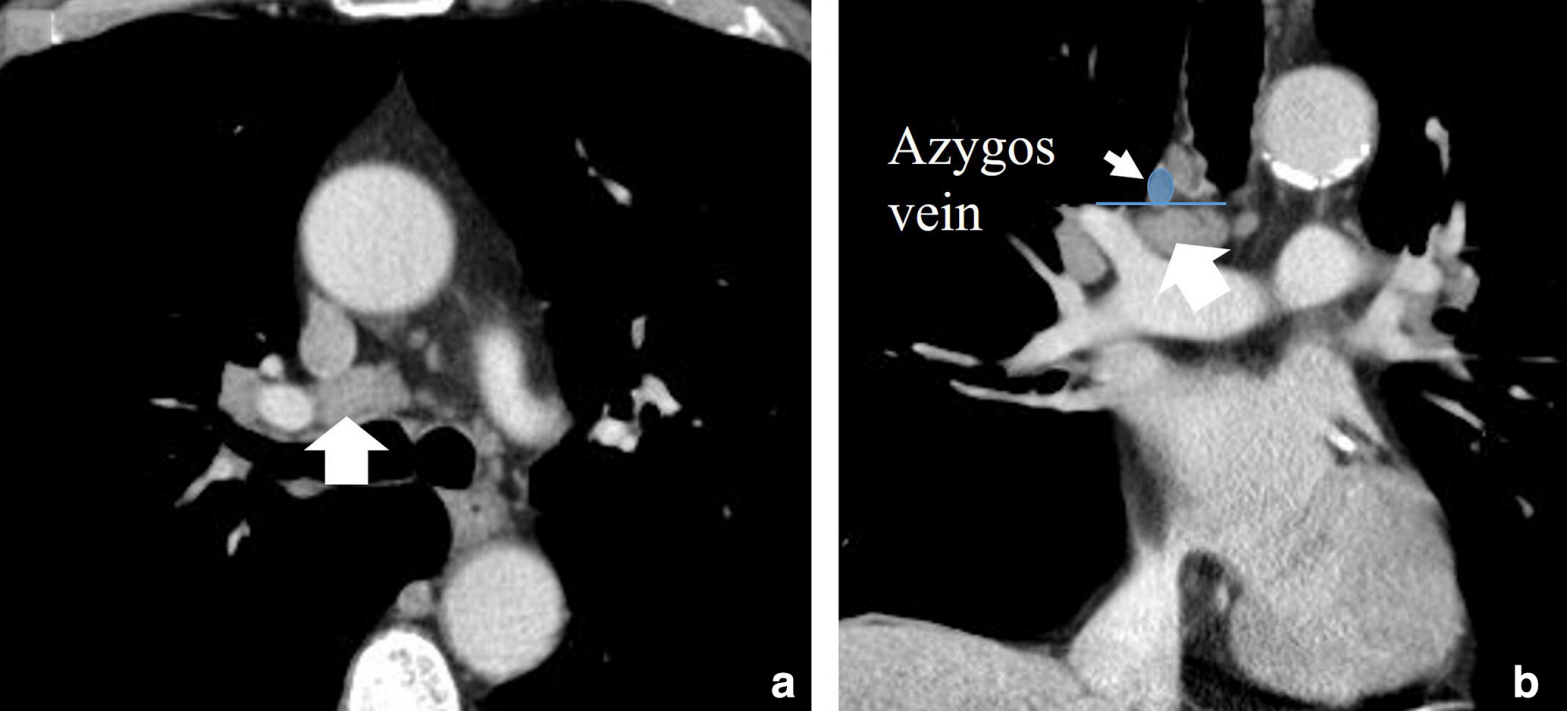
(a) Axial and (b) coronal contrast enhanced CT showing and enlarged (>10 mm) lymph node in the station 10 on the right sight (10R). Please note that the lymph node is below the level of the azygos vein.
